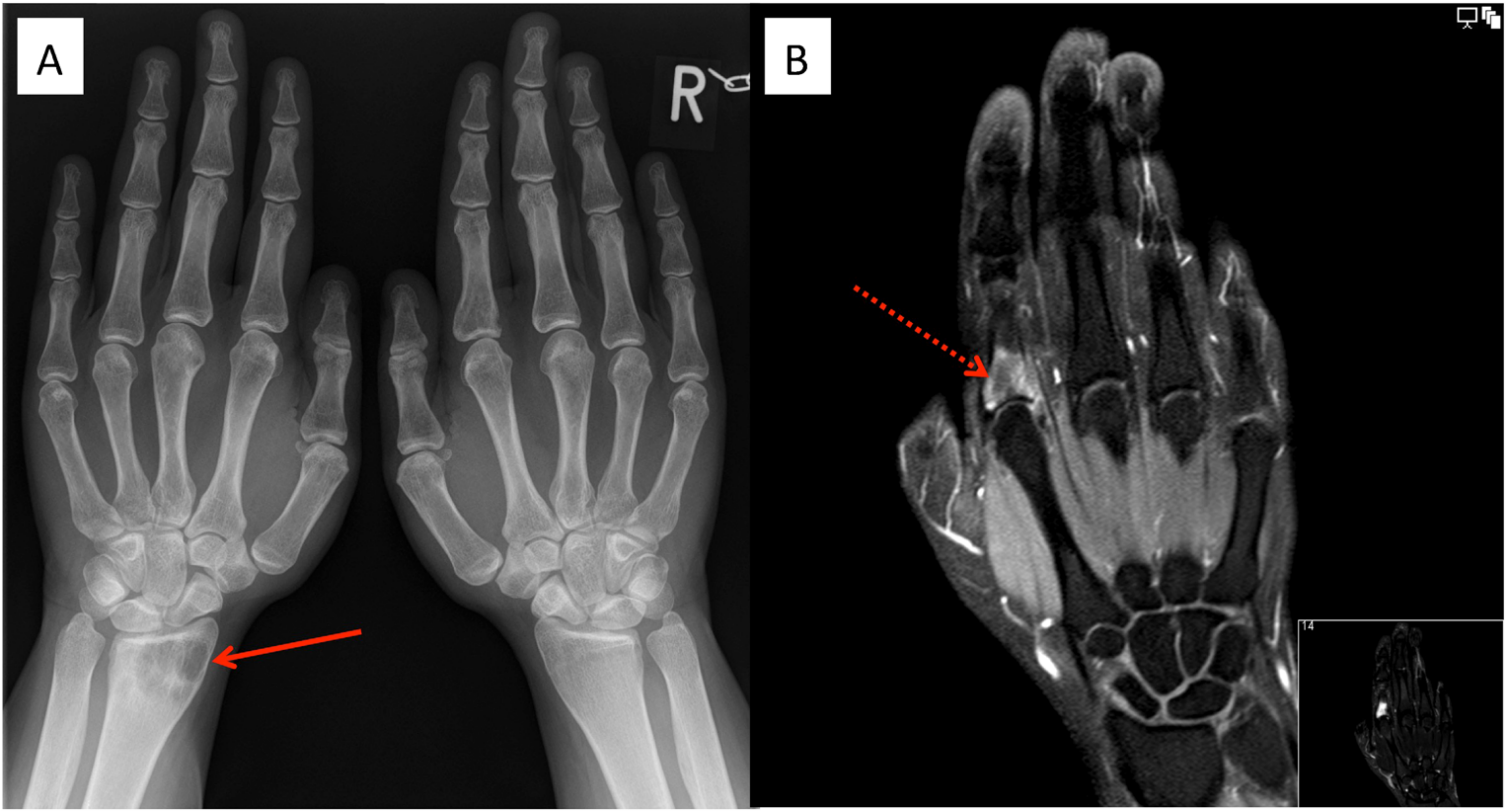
Bone involvement in Rosai-Dorfman disease (RDD). X-ray of the hands shows a lytic lesion (*arrow*) of the left distal radius (a). MRI demonstrated an additional mass lesion (*dotted arrow*) involving the proximal part of the right second phalanx with soft tissue extension (b)
